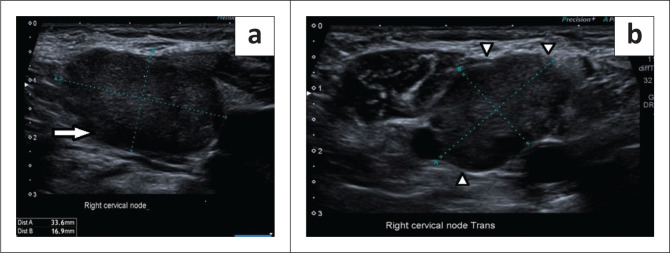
Right cervical pathological lymphadenopathy (a) Longitudinal and (b) transverse ultrasound images demonstrated loss of the fatty hila (solid white arrow), lobulated contours (solid white arrow heads) and increased short axis diameter of 1.69 cm.
